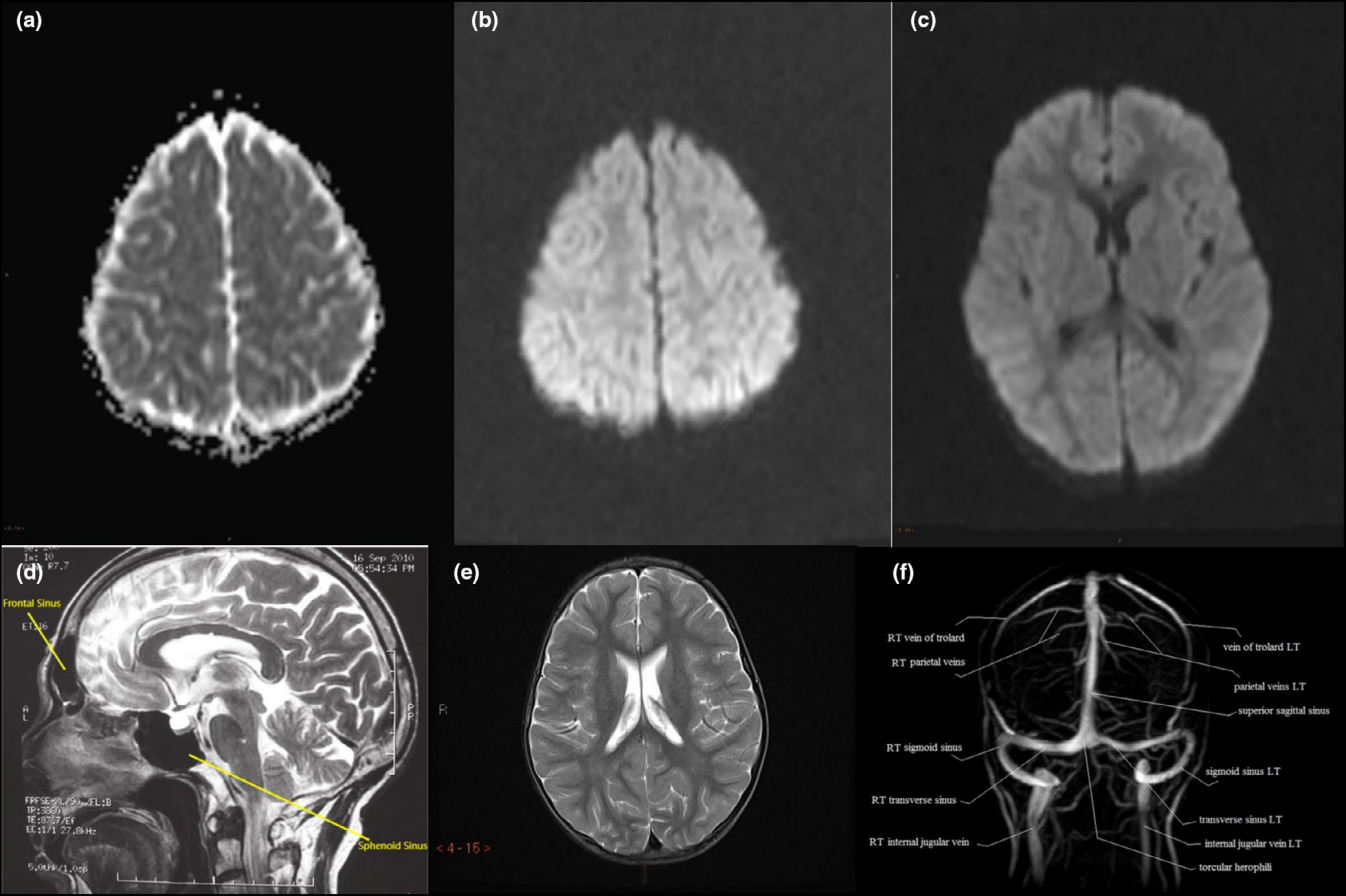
Control images of magnetic resonance imaging (MRI) of the brain. (a) Apparent diffusion coefficient (ADC) showing Frontal Lobe (ADC is a measure of the magnitude of diffusion of water molecules within tissue, and is calculated using MRI with diffusion‐weighted imaging (DWI). (b) Diffusion‐weighted imaging showing Frontal Lobe. (c) Diffusion weighted imaging showing bilateral parietal lobe and globus pallidus. (d) T2 weighted MRI showing frontal (arrows) and sphenoid paranasal sinuses (arrows). (e) T2 weighted MRI showing the periventricular area of the lateral ventricle. T1 and T2 are basic pulse sequences on a MRI. A T1 MRI image supplies information about current disease activity by highlighting areas of active inflammation. A T2 MRI image provides information about disease burden or lesion load (the total amount of lesion area, both old and new). (f) Magnetic resonance angiogram showing dural venous sinuses.
